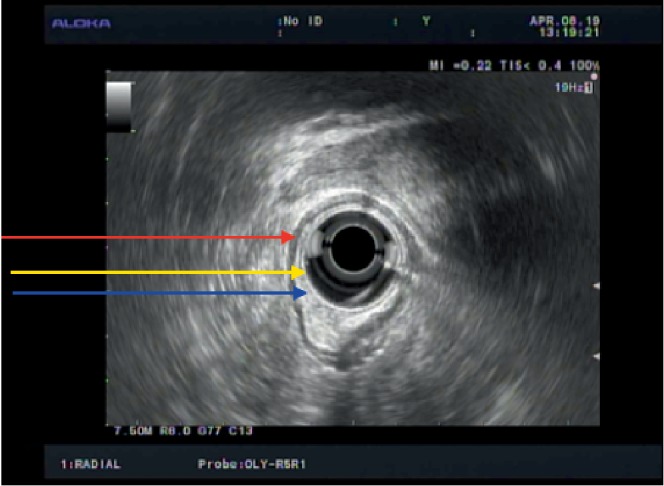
Rectal endoscopic ultrasound (EUS) showing a localized hypoechoic submucosal lesion with tiny hyperechoic bands without muscularis propria invasion. Muscularis mucosae (yellow arrow), submucosa (blue arrow), and muscularis propria (red arrow).
